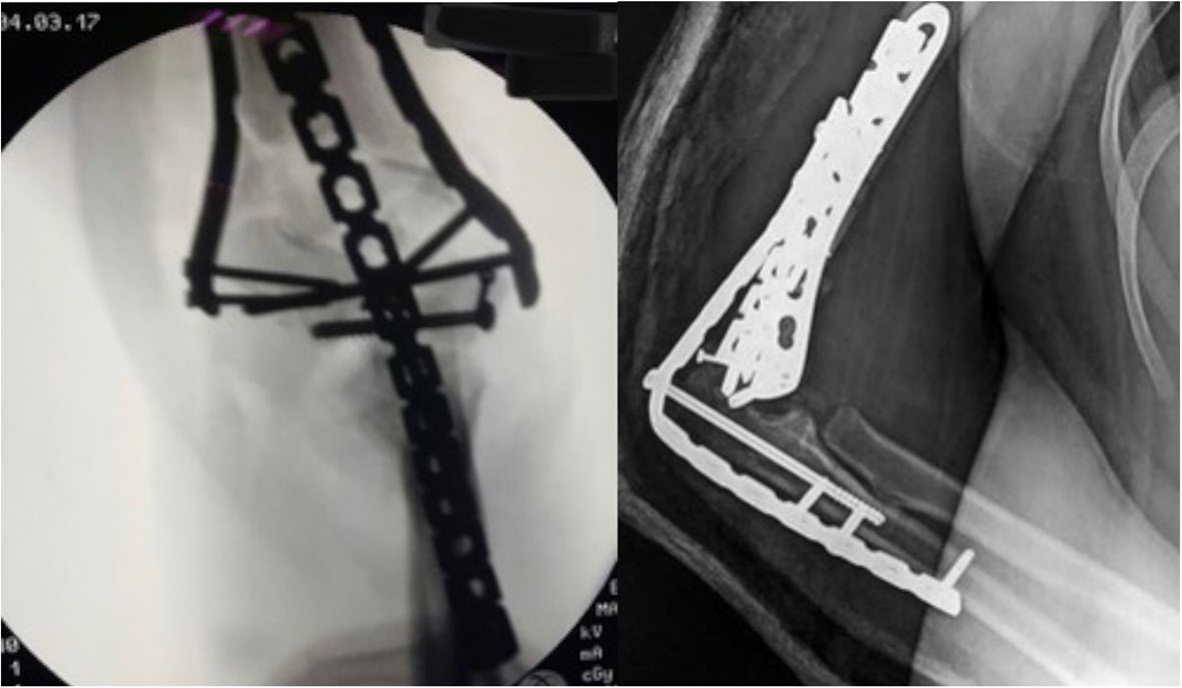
X-rays of the forementioned patient (case #14) fixed with preshaped column plate and augmented with a temporary spanning elbow plate. Olecranon osteotomy in this patient was fixed with an intramedullary screw inserted through the plate
